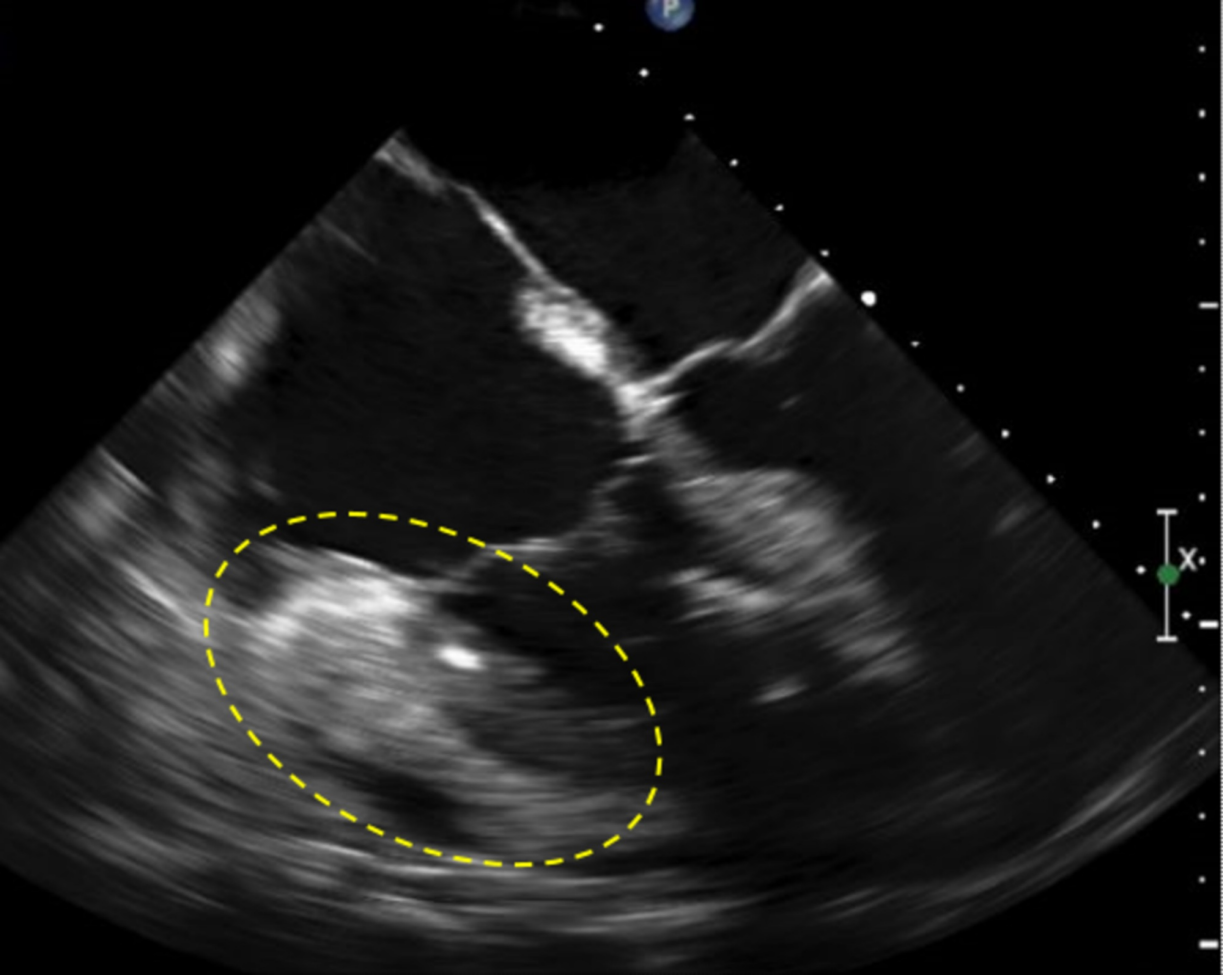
Transesophageal echocardiogram showing maximum RV thickening around the tricuspid annulus (dotted area) RV: right ventricle
